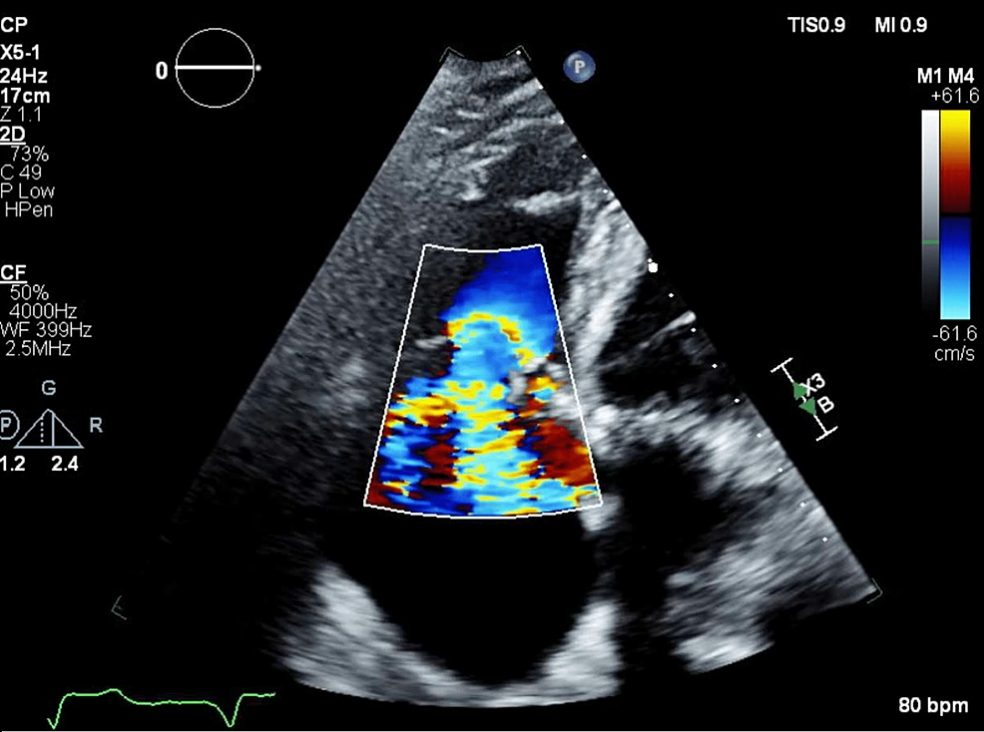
Ultrasonogram showing severe tricuspid regurgitation
